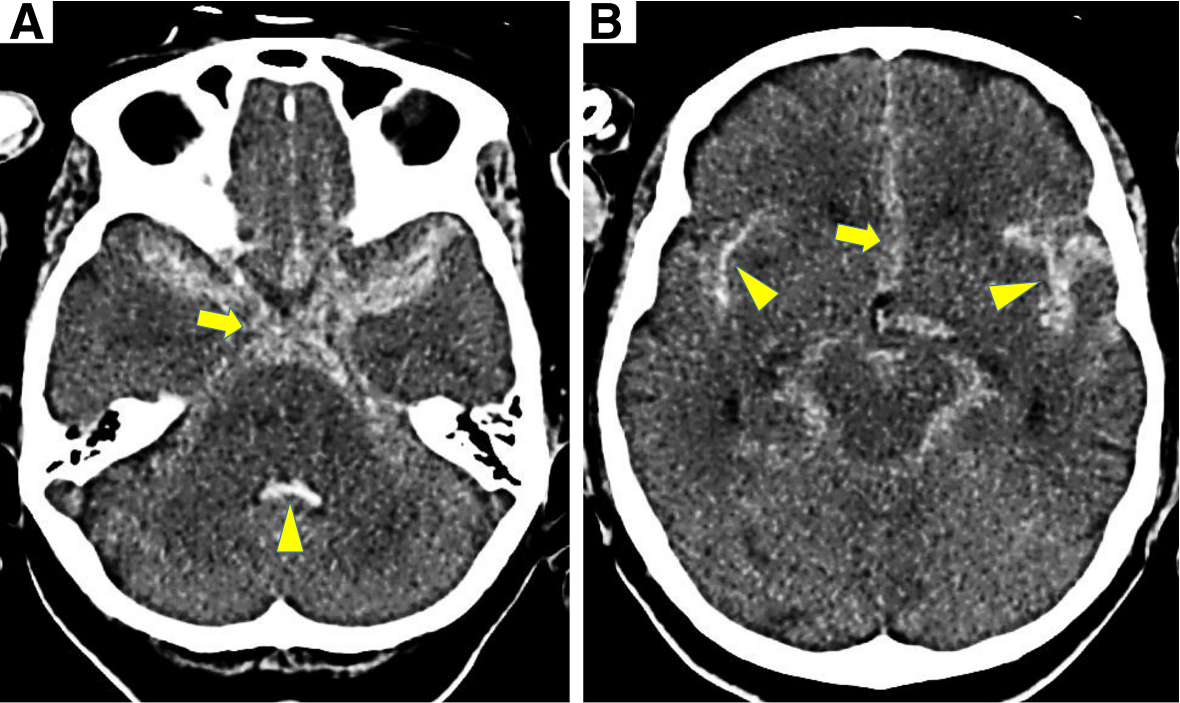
Typical non-contrast CT images of SAH showing blood in the subarachnoid space. The slice is at the level of the suprasellar cistern in a. Blood filling the basal cisterns, and there is hyperattenuating blood in the suprasellar cistern spreading laterally into the medial portion of the Sylvian fissures. In addition, there is blood that has refluxed into the fourth ventricle. An *arrow* indicates the blood in the suprasellar cistern. An *arrowhead* indicates blood in the fourth ventricle. The slice is at the level of the midbrain (mesencephalon) in b. There is blood in the perimesencephalic cistern extending anteriorly into the anterior interhemispheric fissure at the level of the midbrain. Blood extending bilaterally into the Sylvian fissures. An *arrow* indicates the blood in the anterior interhemispheric fissure and the blood in lateral extents of the Sylvian fissures are indicated by *arrowheads*
